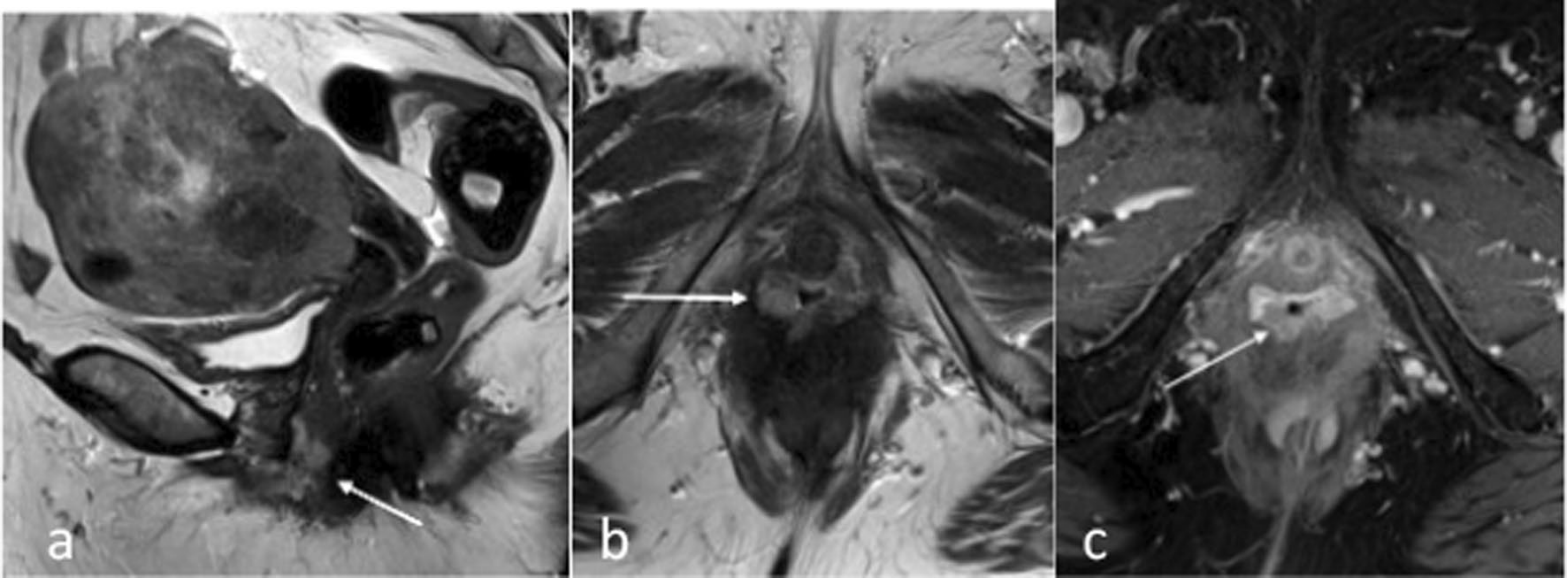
Stage IIIB endometrial carcinoma with vaginal involvement. There is a large tumour with extension through the myometrium into the serosa. On the sagittal (a) and axial (b) T2W MR images, there is a nodule (arrow) with intermediate T2 signal caudally at the level of the vagina on the right, in keeping with a drop metastasis. On axial post-contrast T1W image (c), the drop metastasis is an hypoenhancing lesion (arrow) compared to the hyperenhancing vaginal mucosa and muscular layer. This was diagnosed with biopsies confirming endometrioid carcinoma
